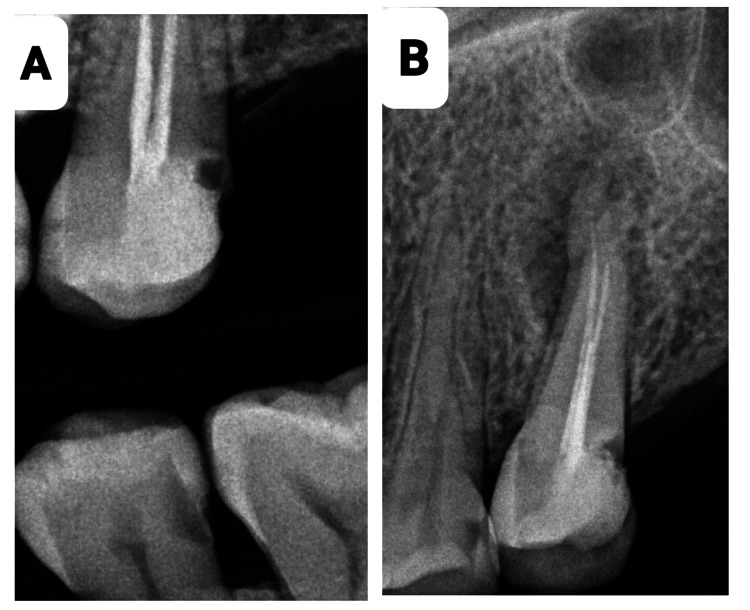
Intraoral bitewing (A) and periapical (B) radiographs show recurrent caries under the restoration and a previous RCT with short obturation and large radiolucency at the root apex.
